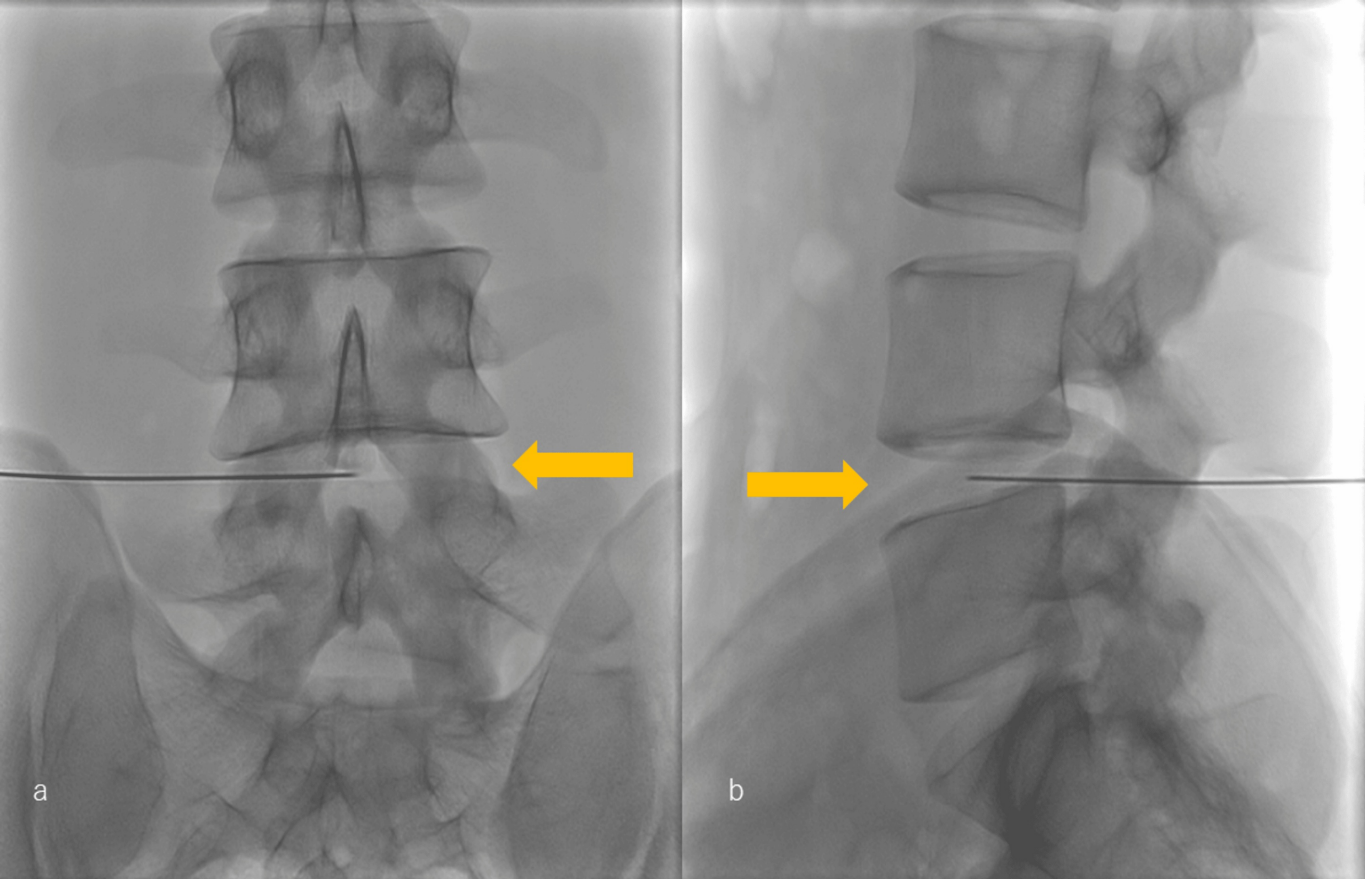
Plain radiographs of the lumbosacral spine during condoliase injection therapy The puncture needle tip is centered in both the (a) anterior-posterior and (b) lateral views.
